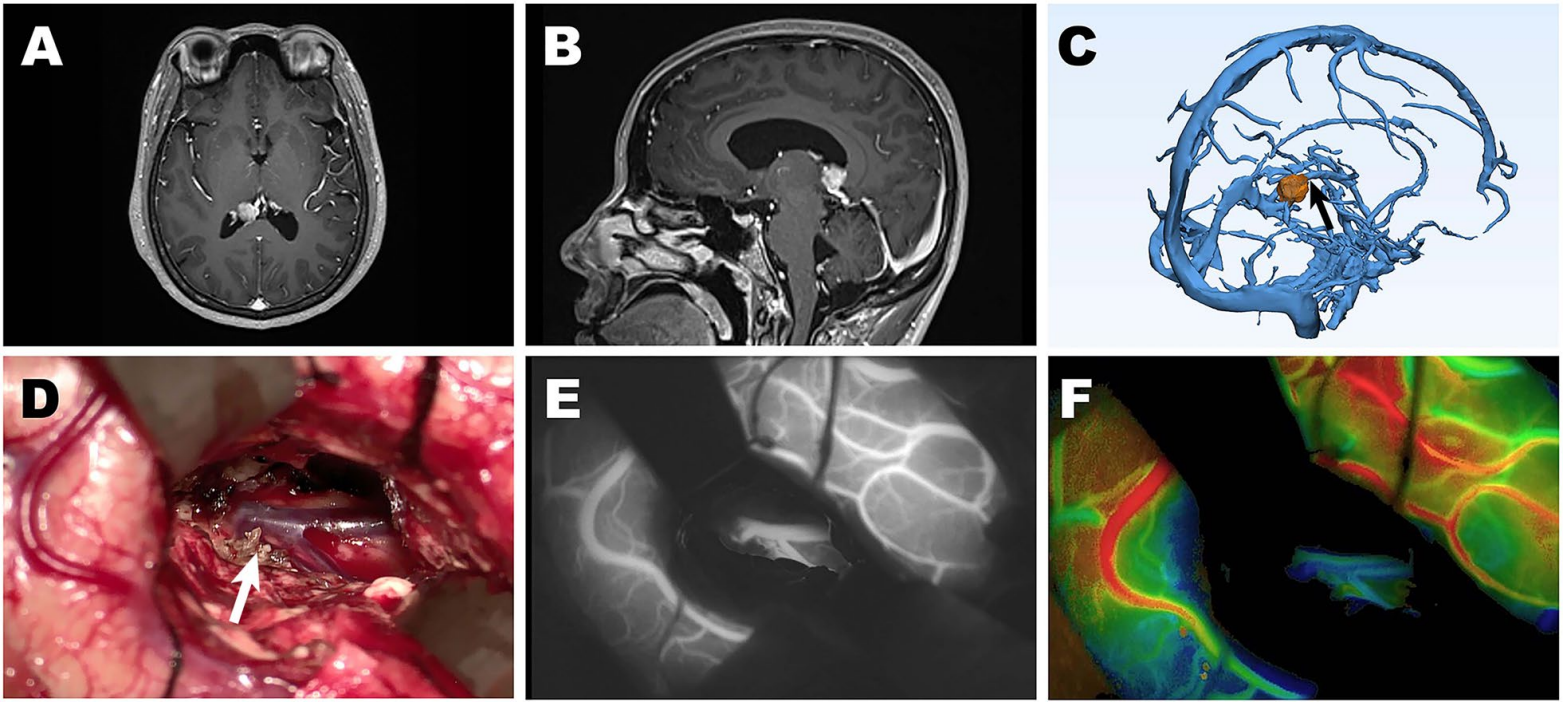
A patient with a mass located at the posterior horn of the right lateral ventricle underwent surgery. A T1- weighted axial and B T1-weighted sagittal MRI with contrast demonstrated a mass located at the posterior horn of the right lateral ventricle. C 3D imaging revealed the involvement of the Galen vein (black arrow) by the tumour (orange). D During the surgical process, the Galen vein (arrow) was preserved. E, F ICG-VA integrated with FLOW 800 clearly demonstrated provided blood flow information of the deep vein
